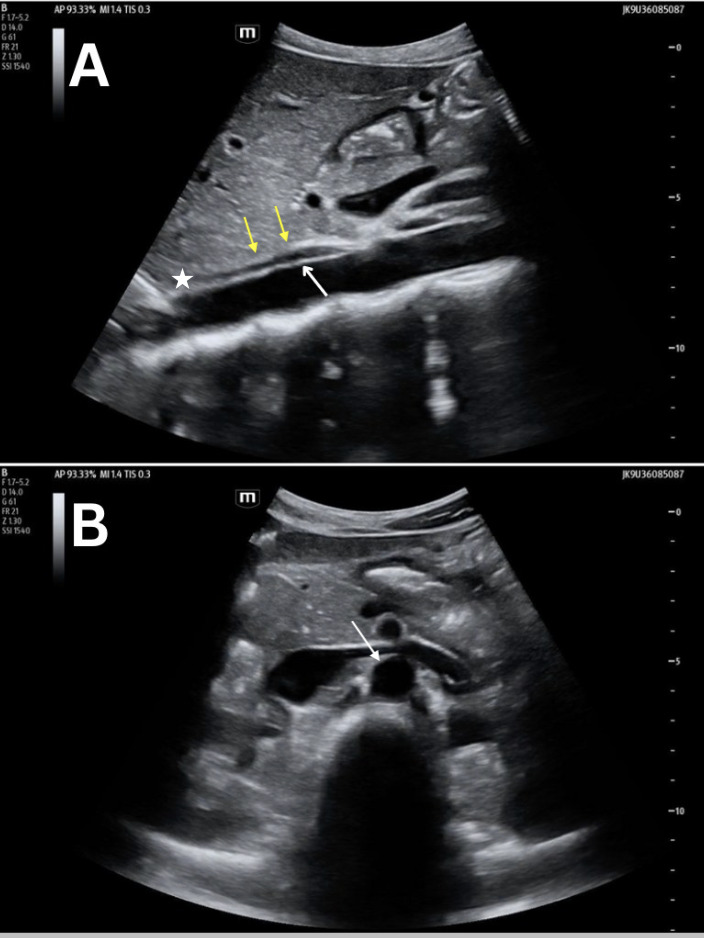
Grayscale point of care ultrasound (POCUS) images of a 25-year-old female standardized patient showing (A) sagittal view with two neighboring linear, hyperechoic structures (double yellow arrow and white arrow) and the intervening hypoechoic crus of the diaphragm (white star) and (B) transverse view with a normal, anechoic lumen of the abdominal aorta (white arrow).
